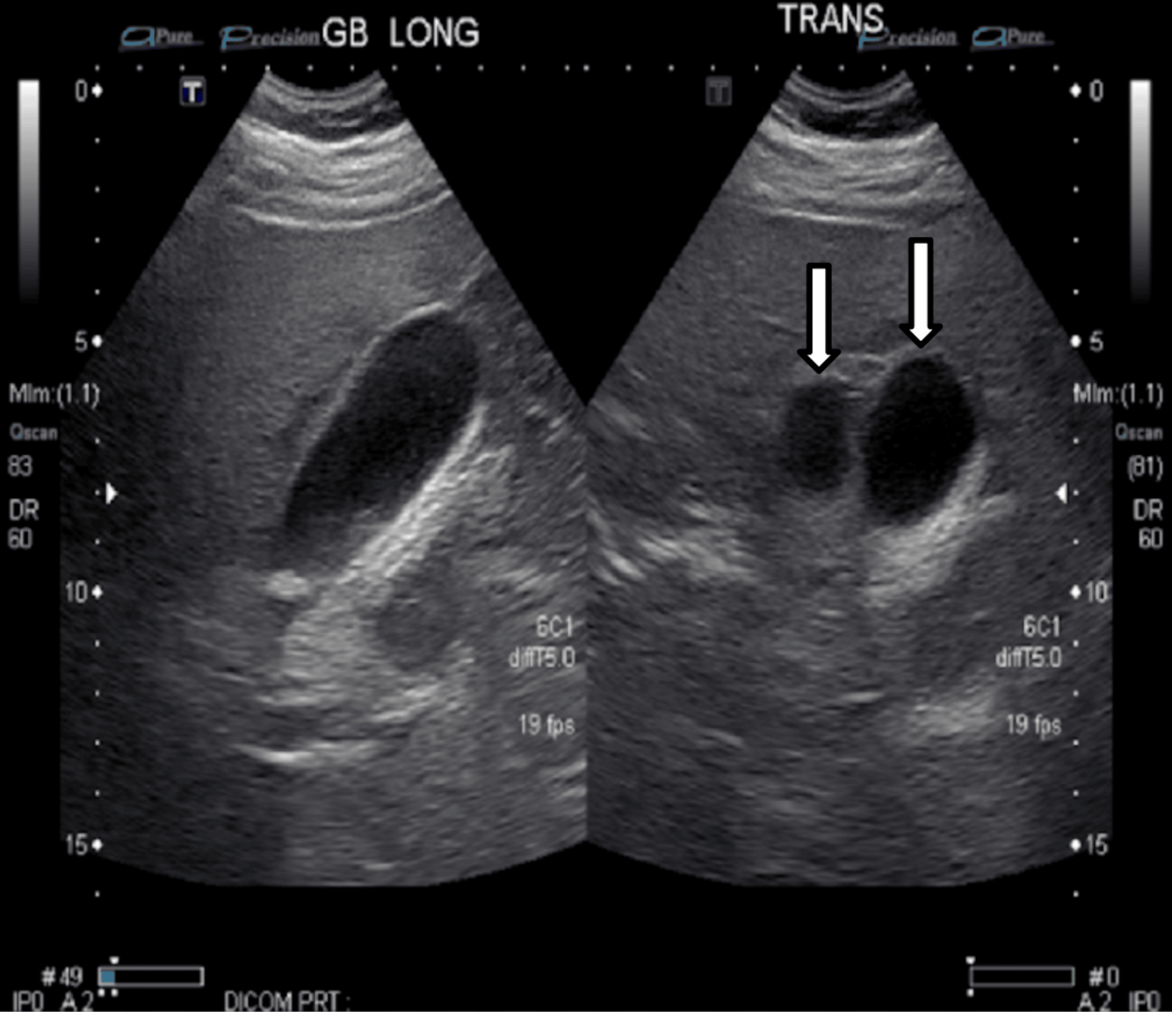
Ultrasound images demonstrating duplicated gallbladder with features of acute cholecystitis Longitudinal (GB LONG) and transverse (TRANS) grayscale ultrasound images of the right upper quadrant demonstrate two distinct anechoic structures consistent with a duplicated gallbladder (as depicted by the white arrows). The walls appear thickened, and internal echoes suggest sludge or debris, supporting a diagnosis of acute cholecystitis
